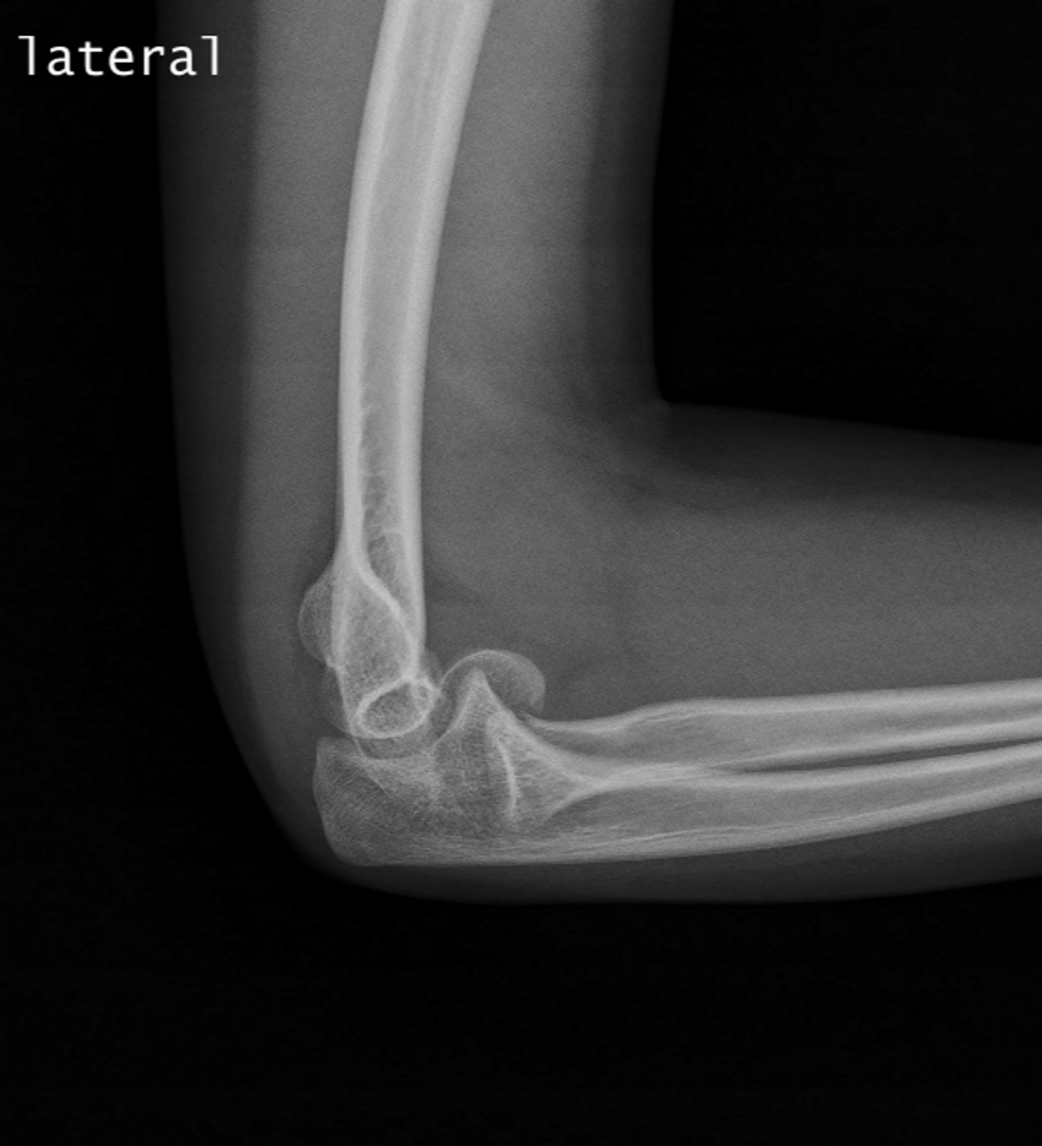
Right Hahn-Steinthal Type 1 capitellum fracture, preoperative lateral radiograph.
